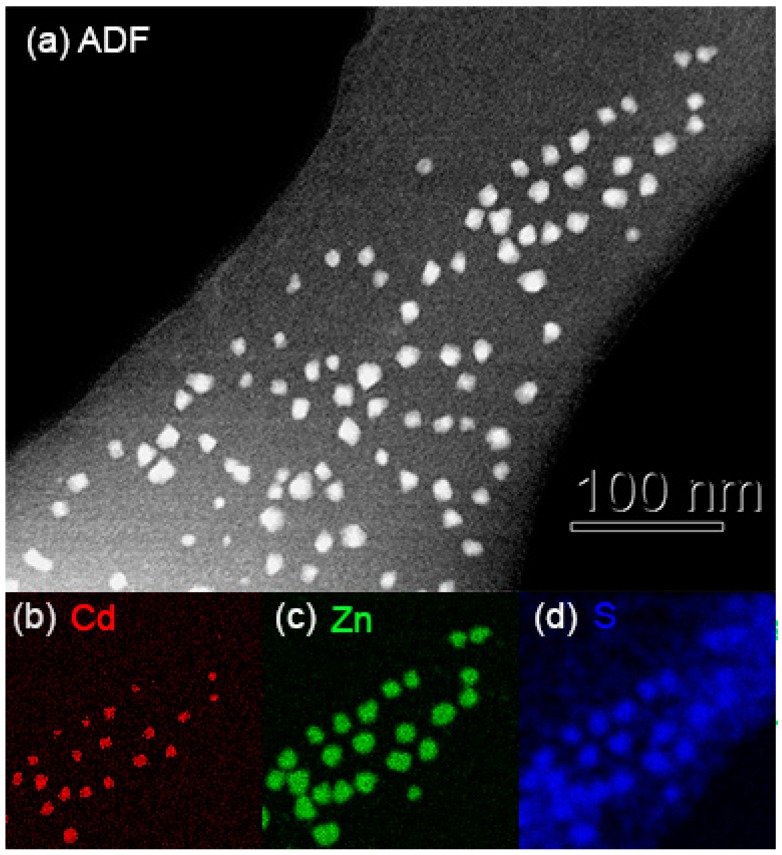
Example of CdZnS colloidal quantum dots with CdS core/ZnS shell structure (a) annular dark-field scanning TEM (ADF-STEM) image of a larger region and (b–(d) X-ray maps of the top right quarter of (a) ~180 nm wide, displaying intensities of (b) Cd_L_ (max = 13 counts), Zn_K_ (max = 63 counts) and S_K_ (max = 58 counts). The weak sulphur background signal in (d) is an artefact due to imperfect background subtraction for the sulphur K-edge at 2.3 keV; also, it suffers from tails of the neighbouring silicon K-edge at 1.8 keV as the supporting carbon film actually contained some silicon.
